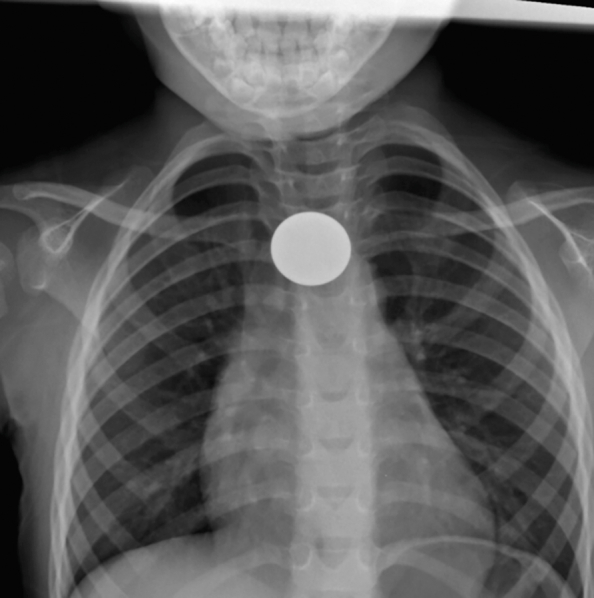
Anteroposterior chest radiograph demonstrating a round radiopaque foreign body overlying the upper mediastinum.
